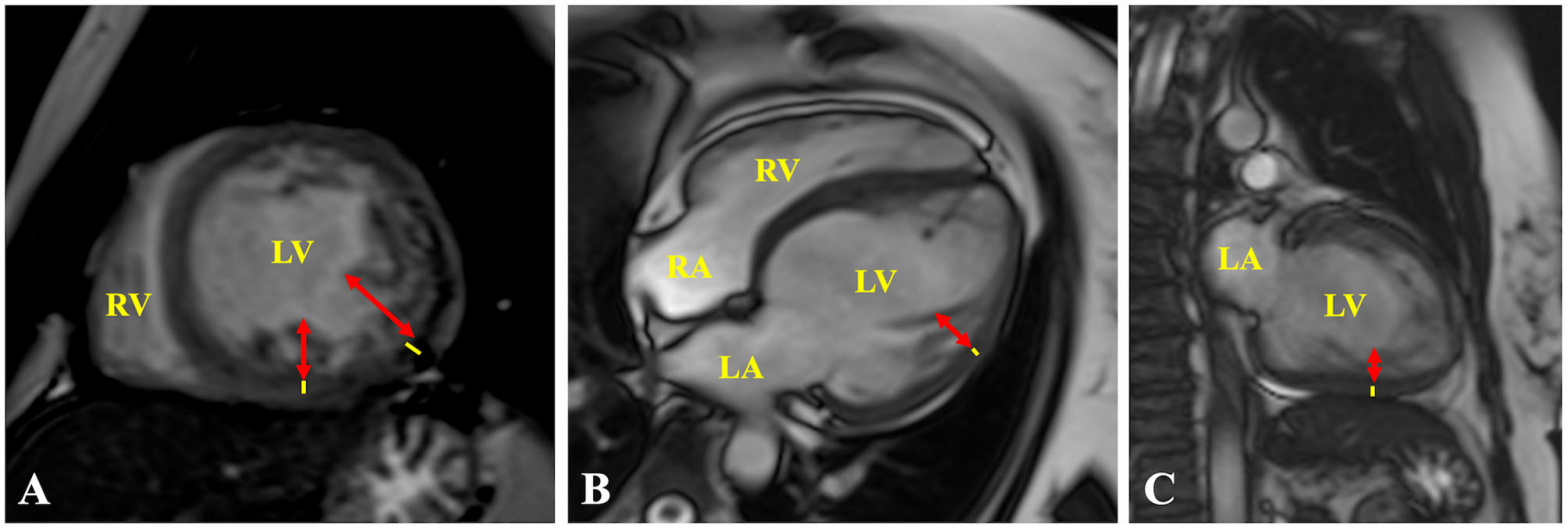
Cardiac MRI. (A) Short axis, (B) 4-chamber, and (C) 2-chamber views showing representative measurements of the ratio of ticker non-compacted (redlines) to thin compacted (yellow lines) myocardium >2.3 at end-diastole, in the (A) mid-inferolateral, (B) mid-anterolateral, and (A,C) mid-inferior segments. LA, left atrium; LV, left ventricle; RA, right atrium; RV, right ventricle.
